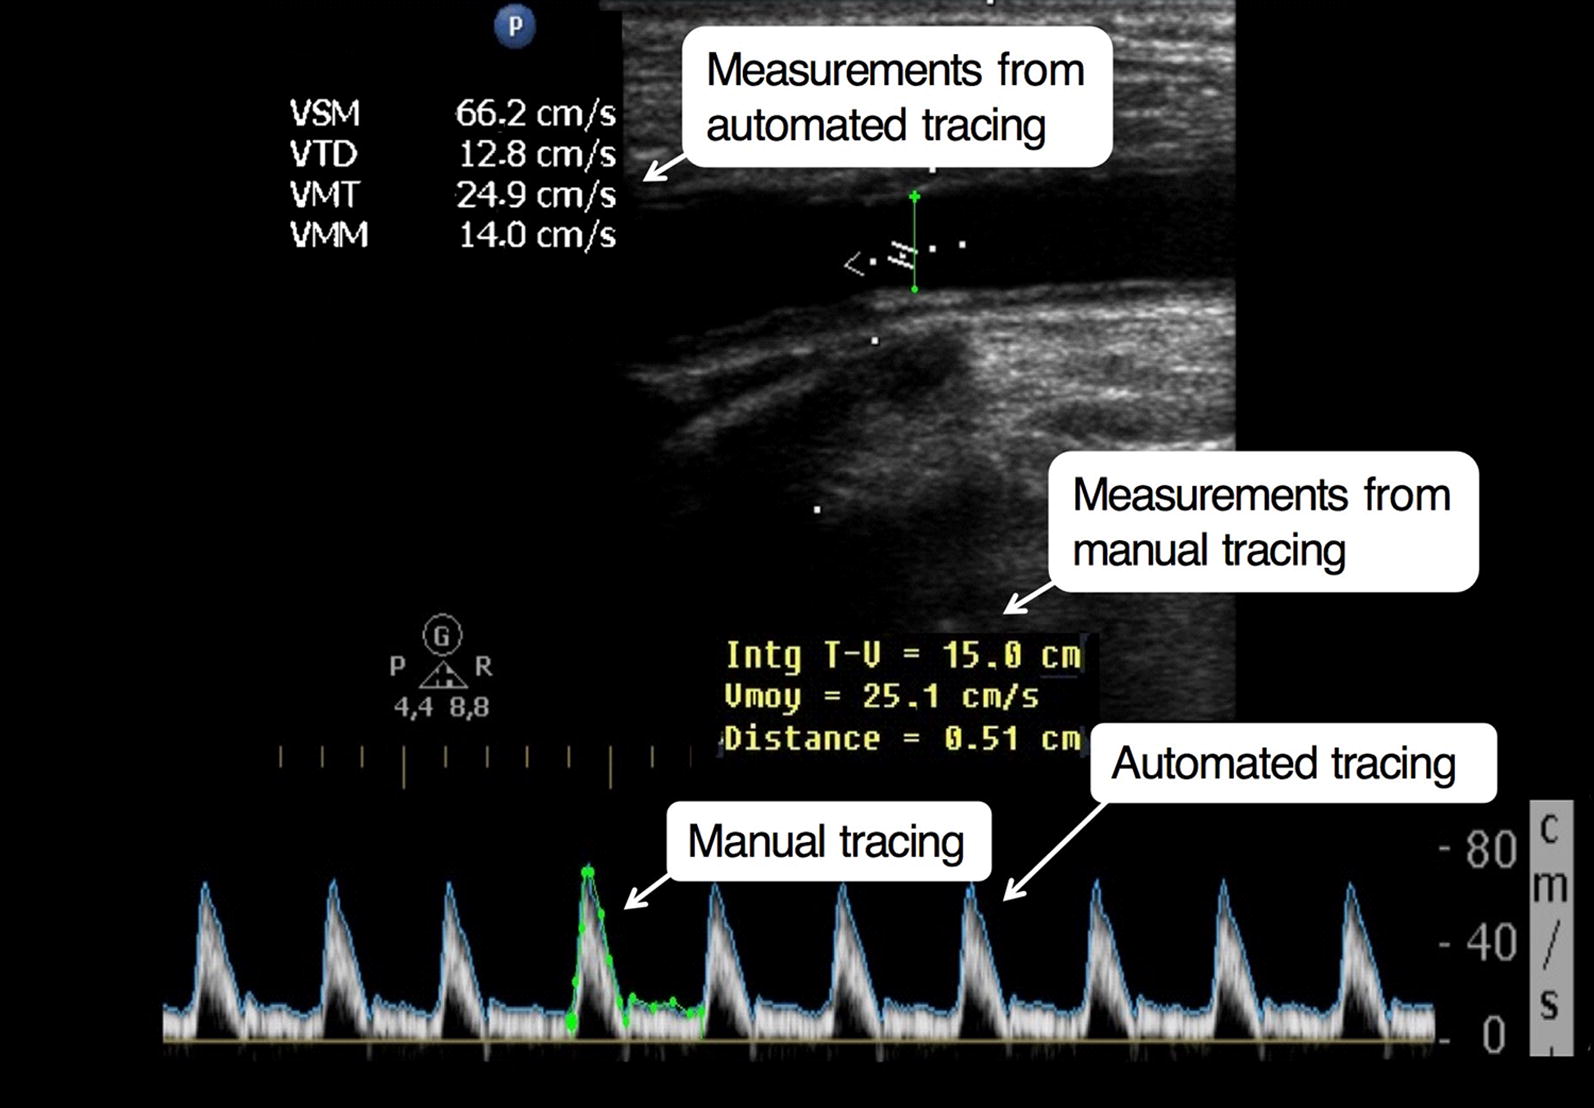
Example of Doppler measurements performed in a patient
